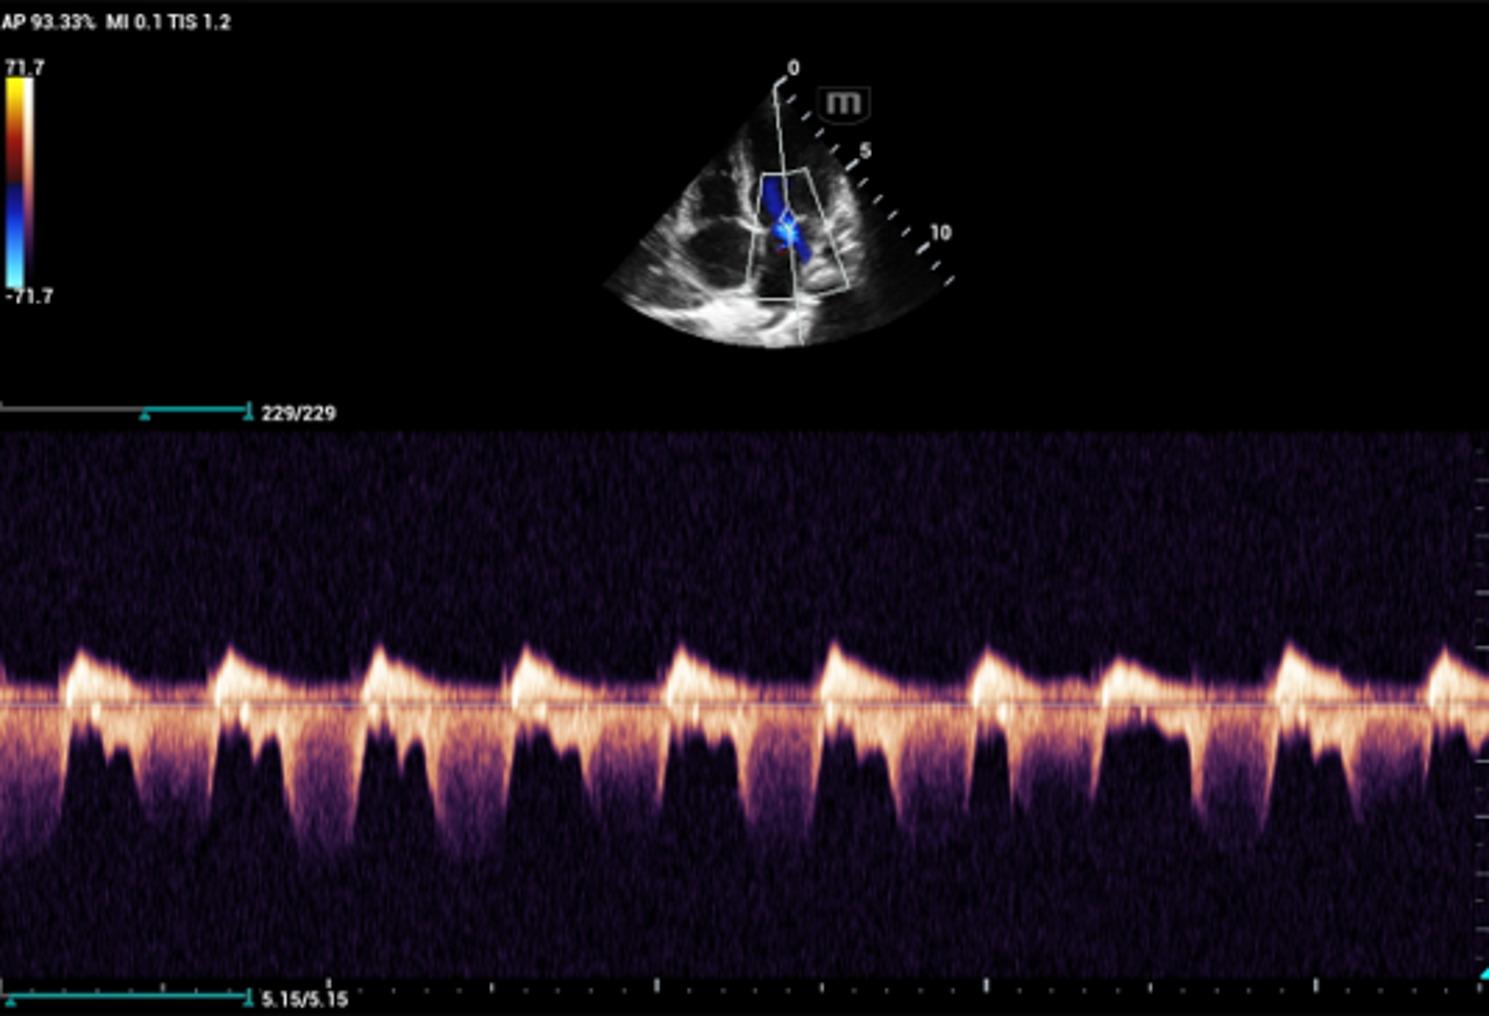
Transthoracic echocardiography (Four-Chamber View): demonstrates functional mitral valve regurgitation secondary to myocarditis
